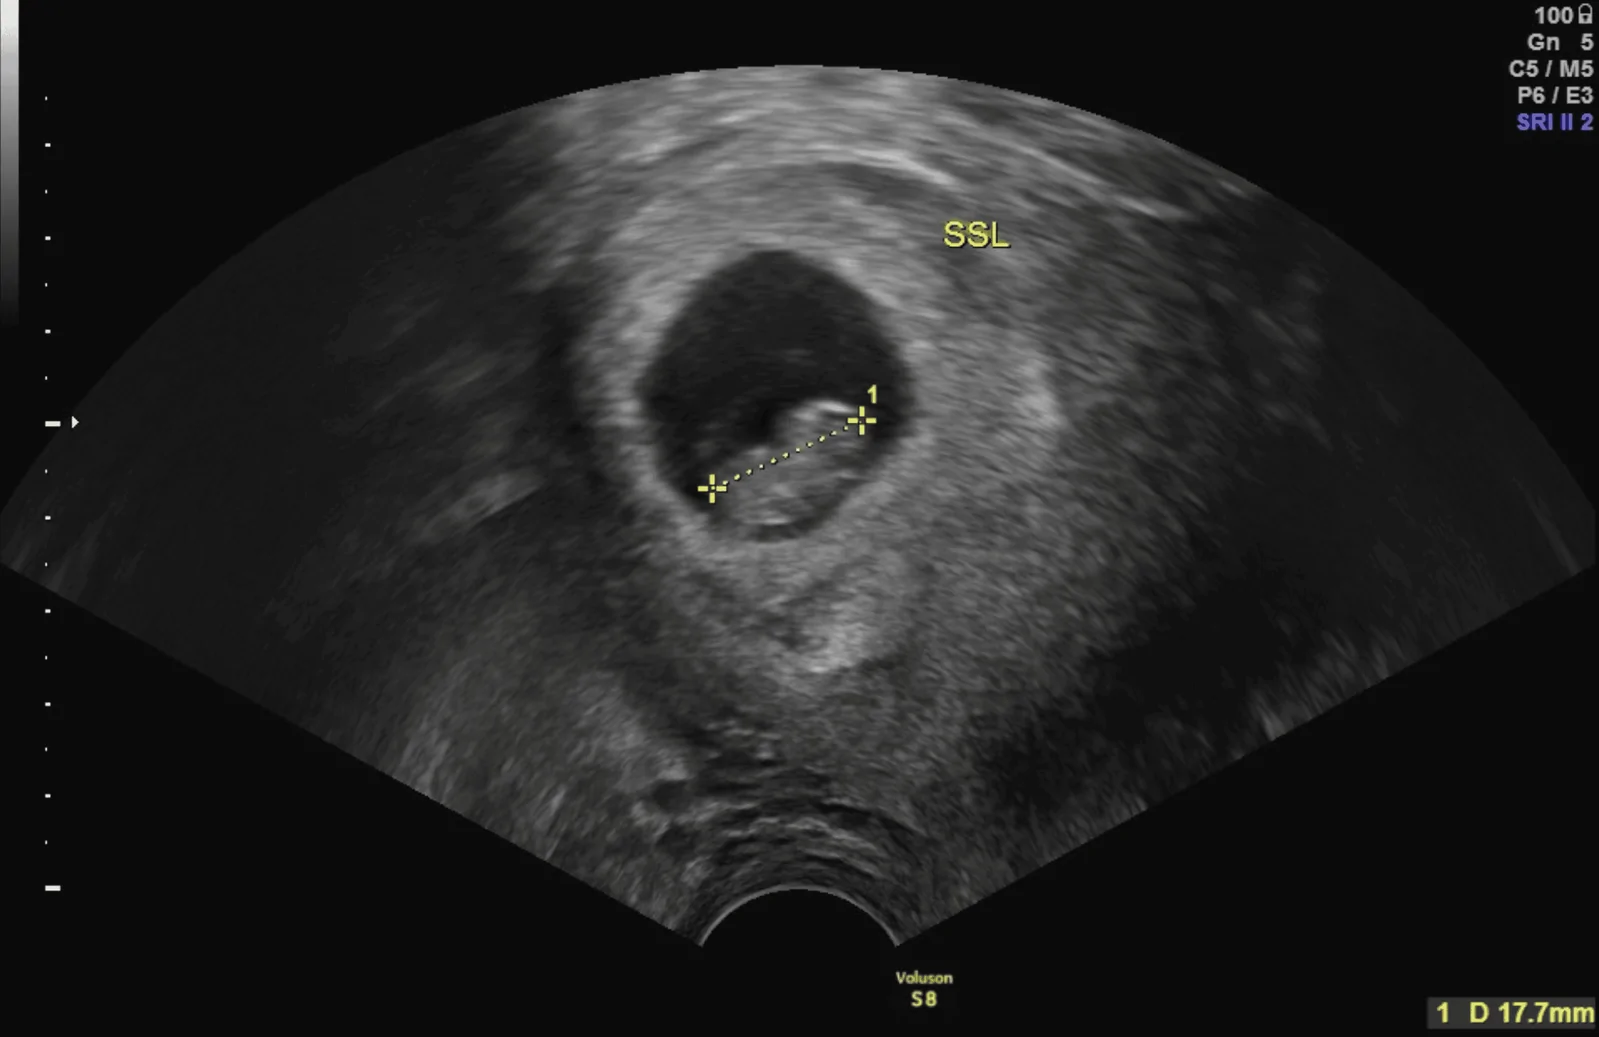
Transvaginal ultrasonography showing an intact intrauterine pregnancy with a crown-rump length (CRL) of 17.7 mm.
